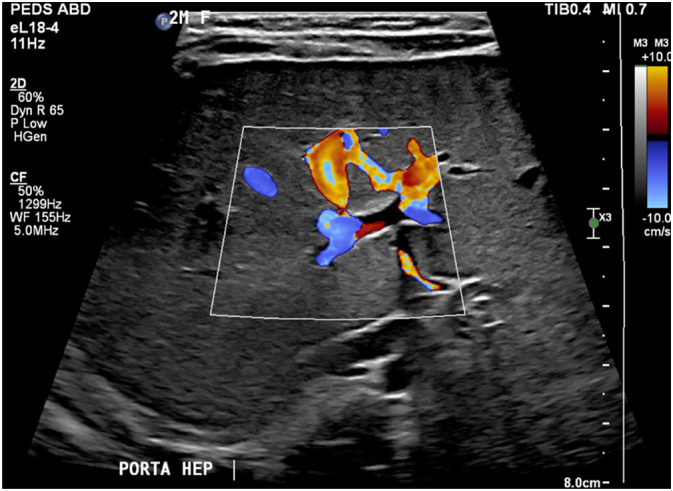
Ultrasound of liver demonstrating patent hepatic vasculature at the porta hepatis.
